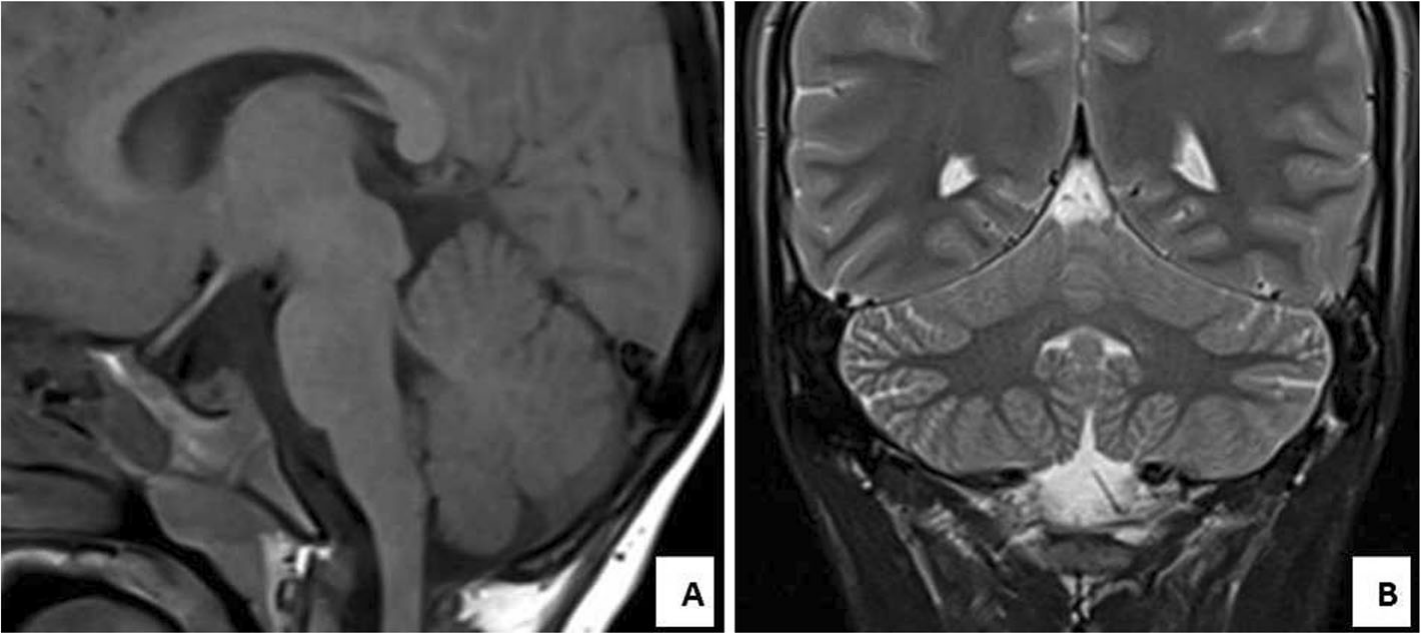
Patient 1 (aged 2.5 years). a Sagittal T1 and (b) Coronal T2-weighted MR images of the cerebellum showing normal cerebellar volume and folia with no signs of atrophy. The vermis has normal size. The cerebellar gray and white matter are also normal
